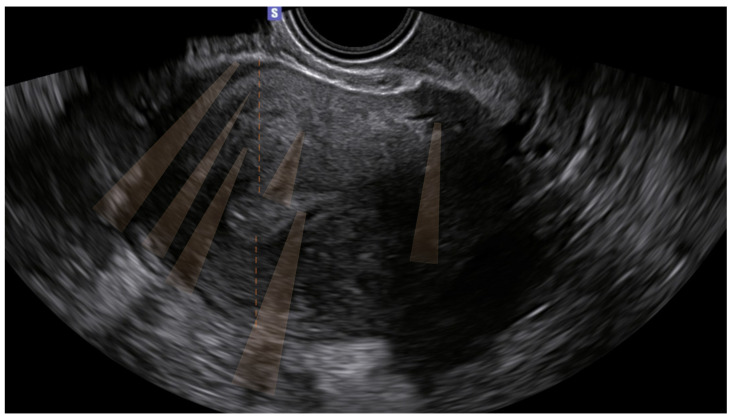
Ultrasound image of the uterus of one of the ten patients who presented only indirect signs (shadowing, highlighted in orange in the figure). The patient was symptomatic (dysmenorrhea).
